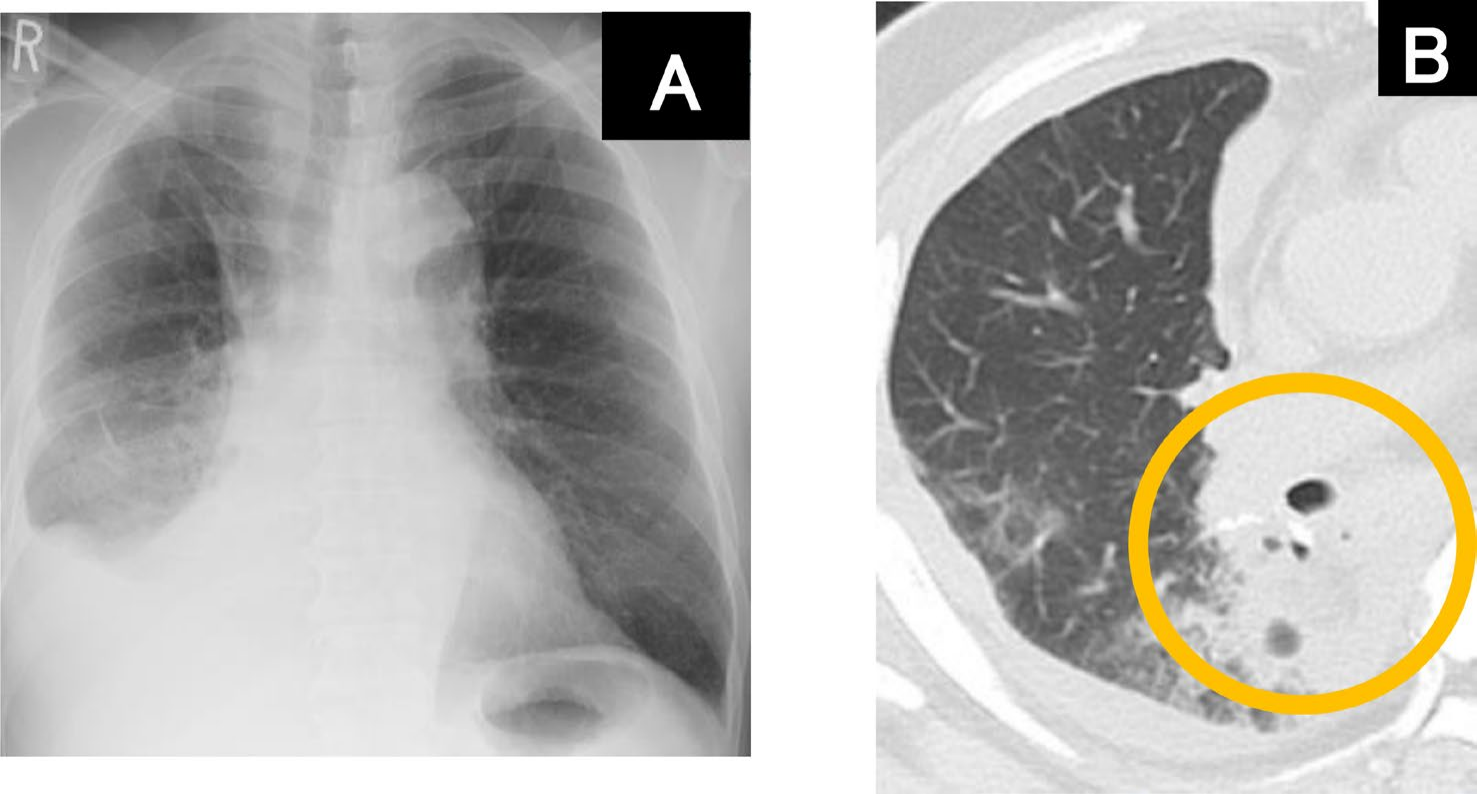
Radiological images on admission (on Day 16). (A) Chest radiograph showing decreased permeability and an infiltrating shadow in the right lower lobe. (B) Chest computed tomography showing an infiltrating shadow with air at the suture in the right lower lobe.
